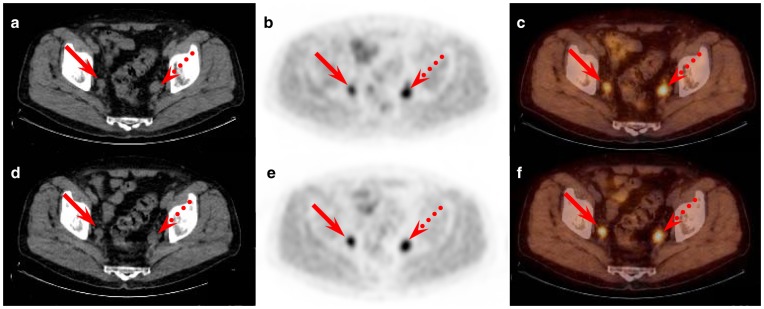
Example of increasing [18F]FCH uptake over time. Example of increasing [18F]FCH uptake over time in a right para-iliac lymph node (red solid arrow; SUV_max_ early: 3.02; SUV_max_ late: 4.35) and a left para-iliac node (red dotted arrow; SUV_max_ early: 3.47; SUV_max_ late: 4.79) of a patient with biological relapse after initially treated prostate cancer. These lymph nodes were classified as malignant. Transversal sections of the Low-dose CT, PET and fused PET-CT images: a, b and c – early phase; d, e and f – late phase.
